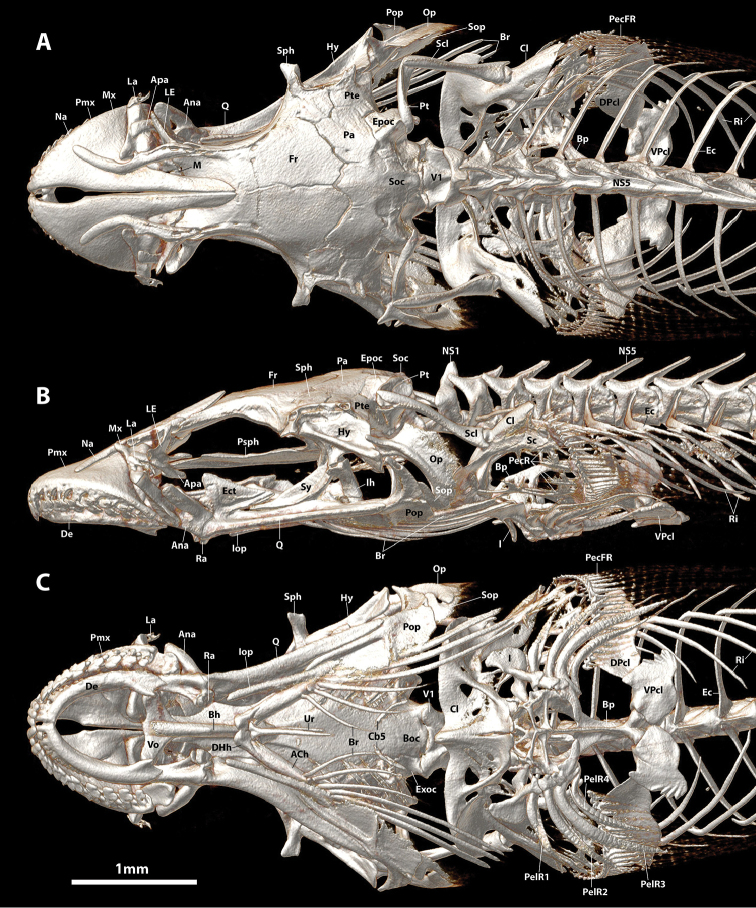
CT scanned anterior skeleton of *Flexorincus*, AIM MA655316, paratype, 23.0 mm SL. A Dorsal view B Lateral view (left side) C Ventral view. Abbreviations: ACh, anterior ceratohyal; Ana, anguloarticular; Apa, autopalatine; Boc, basioccipital; Bp, basipterygium; Br, branchiostegal rays; Cb5, ceratobranchial 5; Cl, cleithrum; DHh, dorsal hypohyal; DPcl, dorsal postcleithrum; Ec, epicentral; Ect, ectopterygoid; Epoc, epiotic; Exoc, exoccipital; Fr, frontal; Hy, hyomandibular; I, pelvic-fin spine; Iop, interopercle; La, lachrymal; LE, lateral ethmoid; M, mesethmoid; Na, nasal; NS1, 5, neural spine of vertebral centrum 1, 5; Op, opercle; Pa, parietal; PecR, pectoral radial; PecFR, pectoral-fin ray; PelFR1-4, pelvic-fin ray 1–4; Pop, preopercle; Pro, prootic; Psph, parasphenoid; Pt, posttemporal; Pte, pterotic; Q, quadrate; Ra, retroarticular; Ri, rib; Sc, scapula; Scl, supracleithrum; Soc, supraoccipital; Sop, subopercle; Sph, sphenotic; Ur, urohyal; V1, vertebral centrum 1; Vo, vomer; VPcl, ventral postcleithrum.
